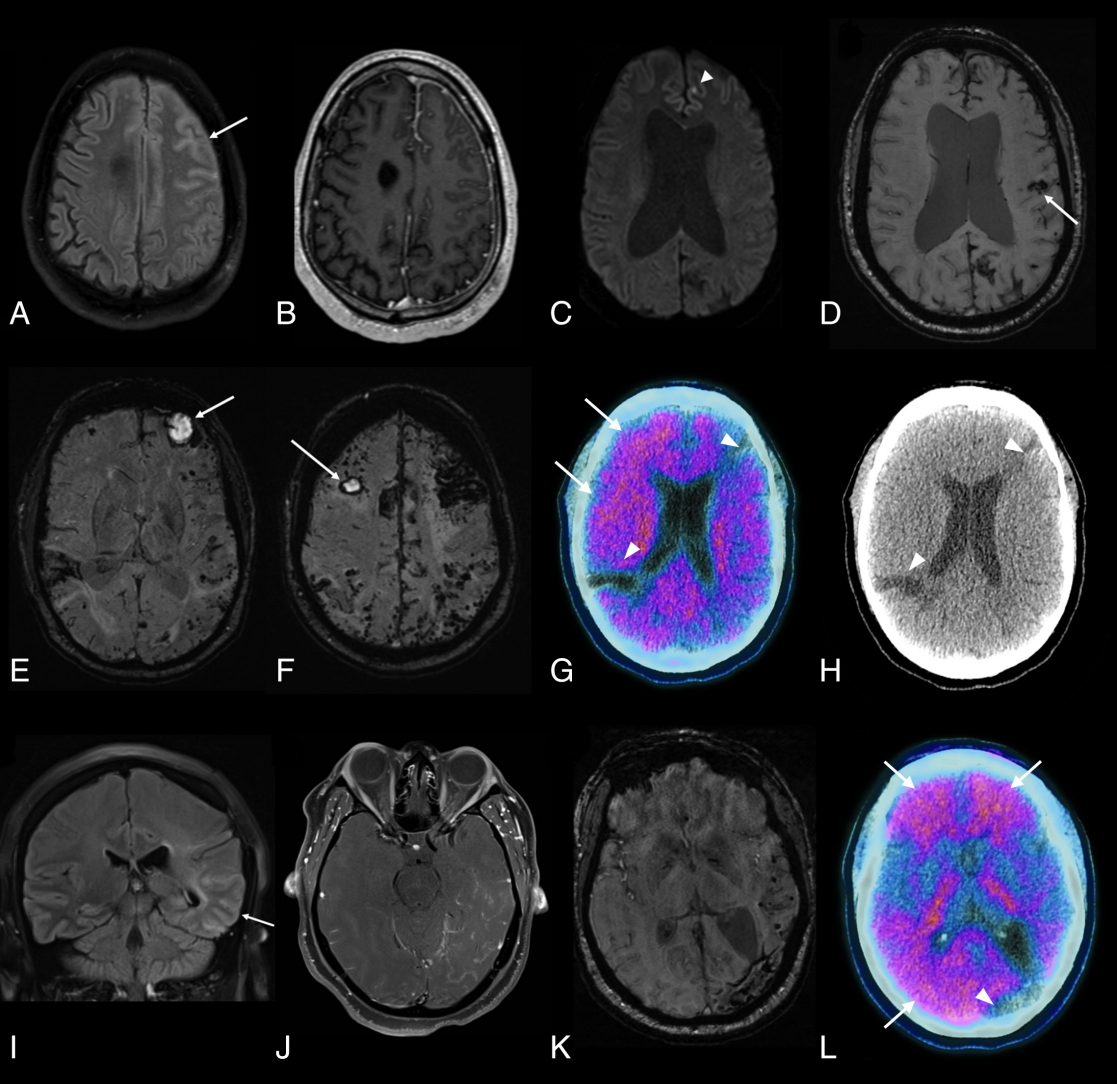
Brain imaging findings. (A–D) Case 1. Fluid‐attenuated inversion recovery (FLAIR; A), T1‐weighted postgadolinium (B), and diffusion‐weighted imaging (C) axial images acquired 2 months after initial presentation demonstrate left frontal gyral swelling and abnormal sulcal signal (*arrow* in A) with leptomeningeal enhancement (B) and a punctate focus of restricted diffusion *(arrowhead)*. (D) Susceptibility‐weighted imaging 4 months later shows a lobar hemorrhage *(arrow)*, multifocal superficial siderosis, and multiple microbleeds. The ventriculomegaly observed in C and D is longstanding (since the choroid plexus papilloma). (E–H) Case 2. (E, F) Susceptibility‐weighted imaging shows hematomas in both frontal lobes *(arrows)* and widespread superficial siderosis and microbleeds, visible as a band of low signal following the leptomeningeal surface of the brain and peripheral, punctate foci of low signal, respectively. (G) ^18^F‐Florbetapir positron emission tomographic (PET)–computed tomographic (CT) imaging shows increased uptake in frontal and parietal cortex (standardized uptake value [SUV] range = 0.0–2.8), suggestive of amyloid deposition *(arrows)*. The 2 areas without uptake *(arrowheads)* correspond with areas of old hemorrhage, as seen on axial CT (H). (I–L) Case 3. FLAIR coronal (I) and T1‐weighted postgadolinium axial (J) images 15 months after initial presentation showing abnormal gyral and sulcal signal in the left temporal lobe *(arrow)* with associated leptomeningeal enhancement. (K) Susceptibility‐weighted imaging 5 months later showing hemosiderin deposition due to previous lobar hemorrhage and numerous temporal microbleeds. (L) Merged ^18^F‐florbetapir PET‐CT imaging shows increased uptake (SUV range = 0.0–3.4) in frontal and posterior (parietal and occipital) cortical regions *(arrows)*; the area with reduced uptake *(arrowhead)* corresponds to the area of old hemorrhagic damage. [Color figure can be viewed at www.annalsofneurology.org]
